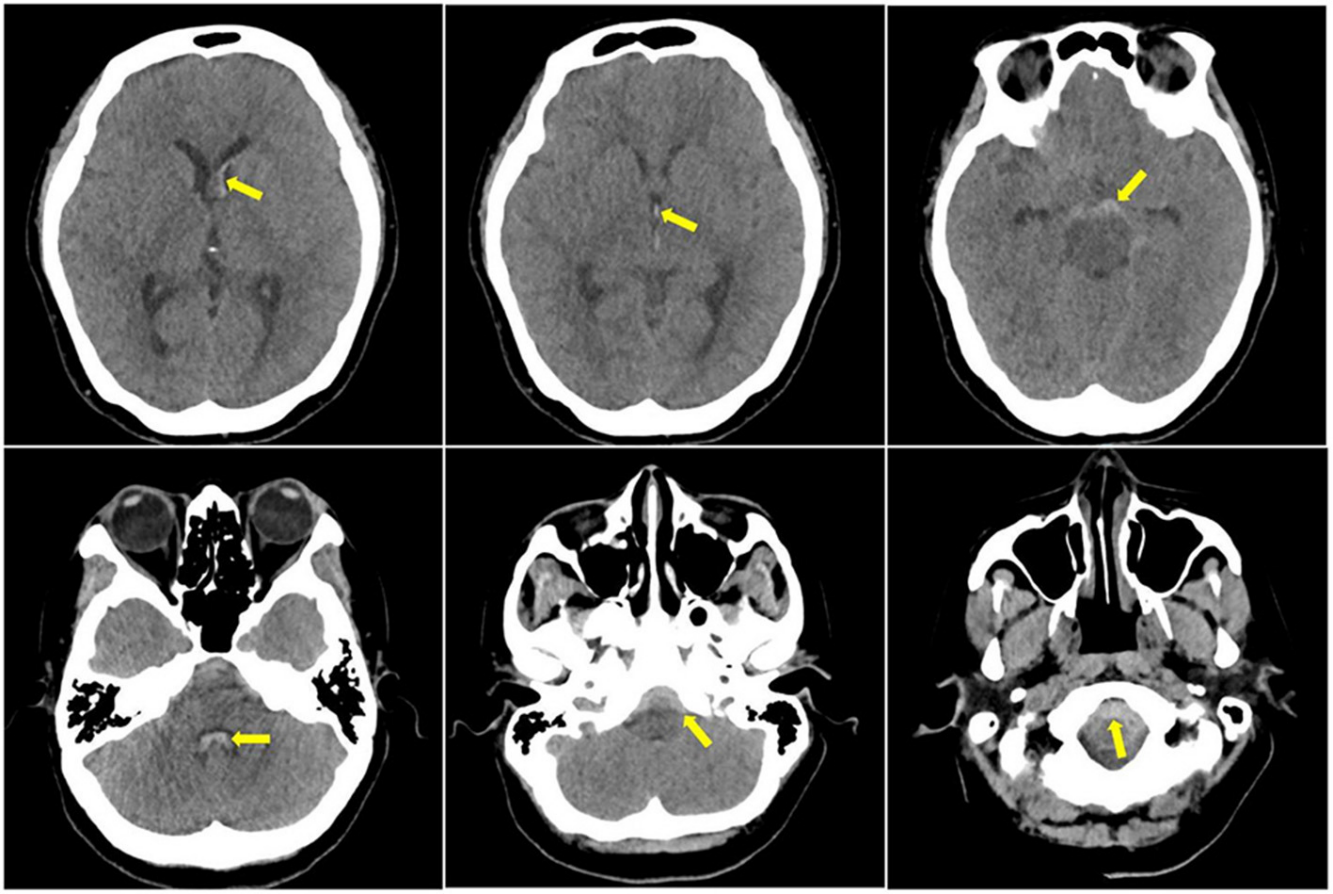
Initial CT head showed subarachnoid haemorrhage (SAH) present in the frontal horn of the left lateral ventricle, third ventricle and fourth ventricle, and the foramen magnum; yellow arrows indicate blood component in the scans.
